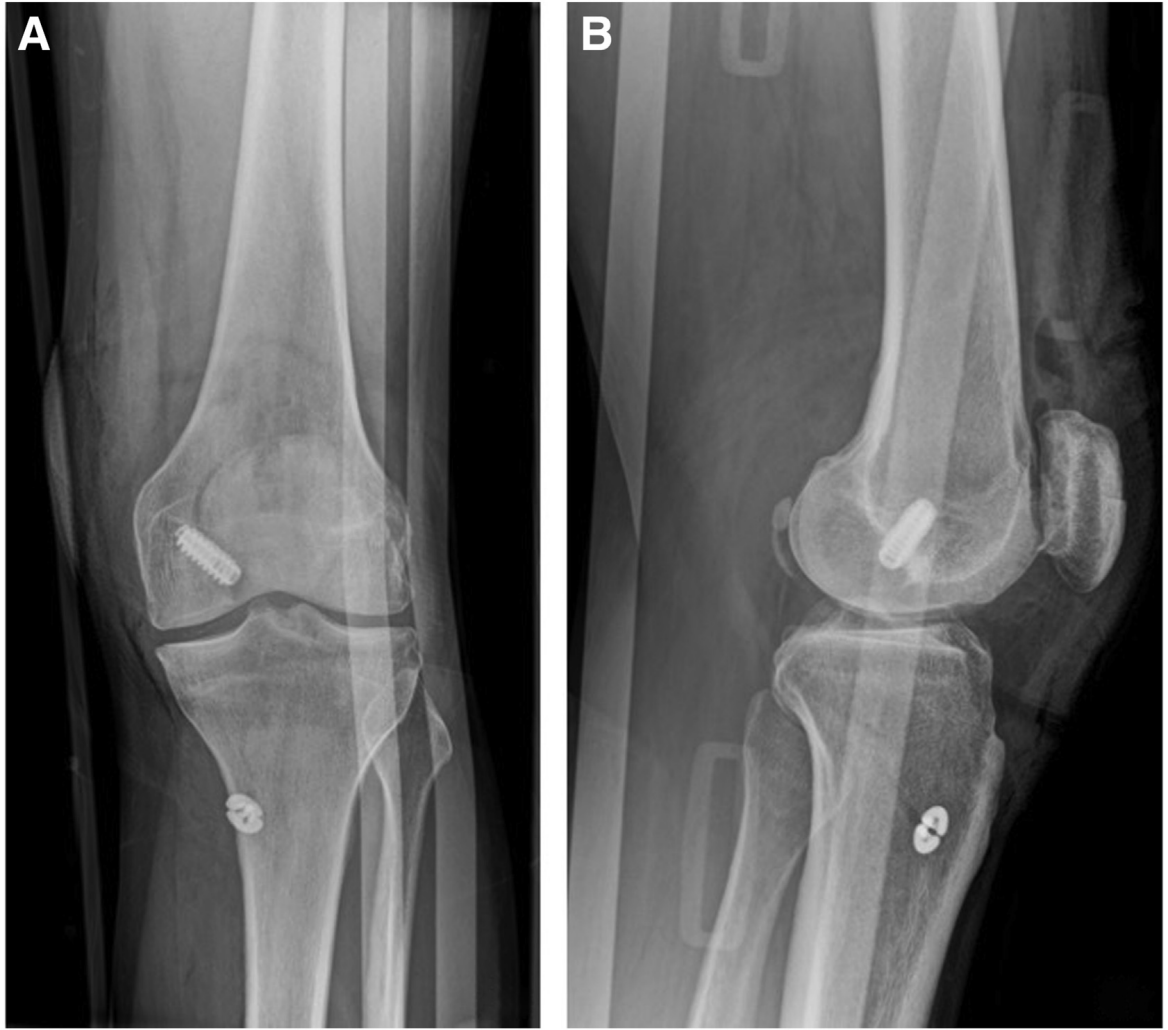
Postoperative radiograph; knee brace was already fitted. The radiograph shows no intra-articular exposition of the fixation device. (A) Anteroposterior view, (B) Lateral view.
